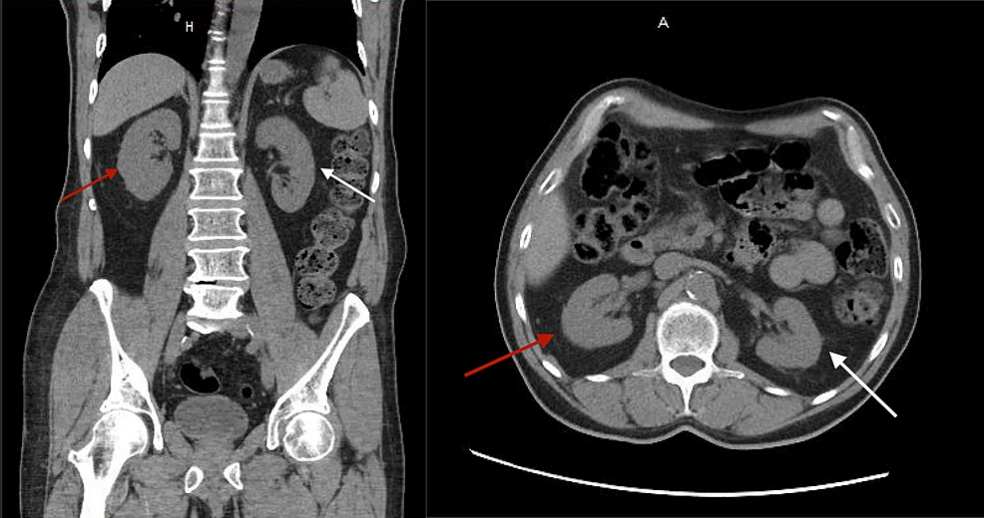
Non-contrast CT coronal view (A), axial view (B) of the abdomen. Figures 1A and 1B show right (red arrow) and left (white arrow) kidneys with no nephrolithiasis or perinephric fat.
